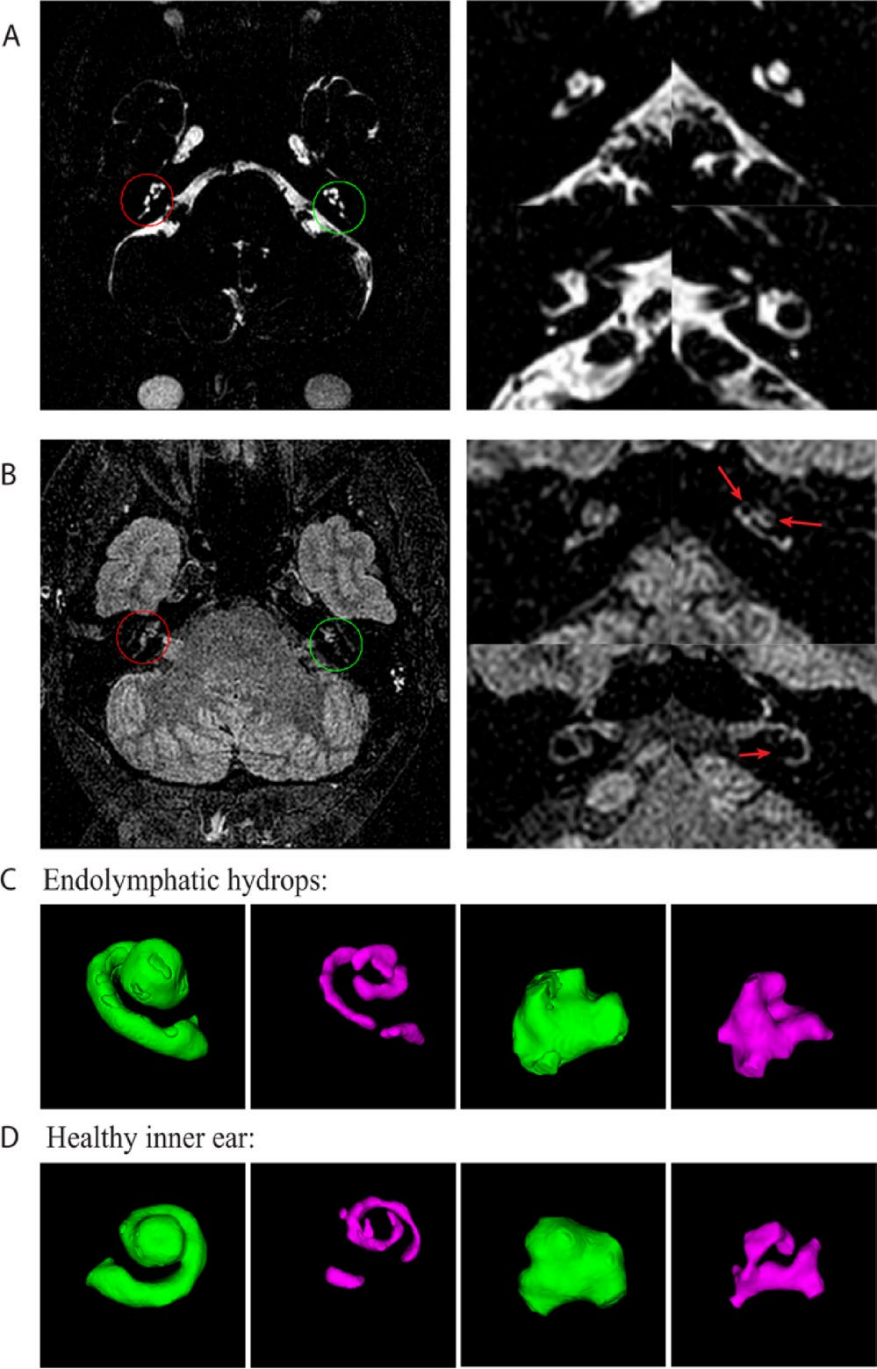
Example CED-FLAIR image from a patient with unilateral EH in the left cochlea and vestibule. (A) STIR images showing hyperintense total fluid. Image on the left shows an axial view of the cranium with inner ear structures circled in magenta (right ear) and green (left ear). On the right, enlarged images are shown of the cochlea in the top row and vestibule in the bottom row. (B) Corresponding FLAIR images to those in panel A in which perilymph only is hyperintense. Left FLAIR image shows axial view of the cranium with inner ear structures circled in magenta (right ear) and green (left ear). Enlarged images are shown on the right of the cochlea in the top row and vestibule in the bottom row. Presence of EH in the left ear is indicated by the enlarged dark areas within the cochlea and absence or thinning of perilymph signal behind the vestibule (red arrows). (C) Example images of hydropic inner ear 3D reconstructions are shown in panel C for the total volume in green and endolymph in magenta of the cochlea (left) and vestibule (right). (D) 3D reconstruction examples of inner ear structures from a healthy volunteer.
